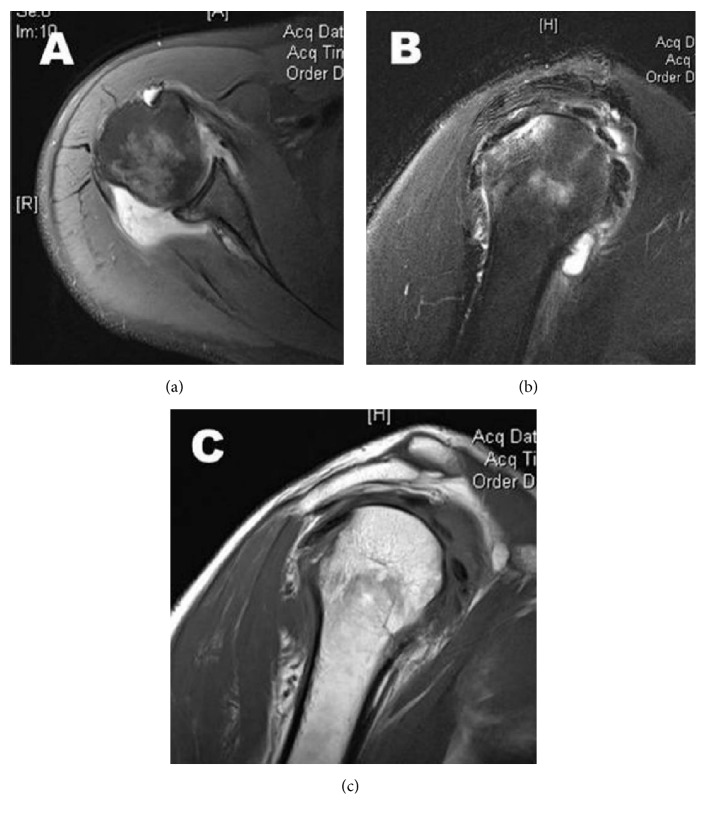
MRI of the right shoulder. (a) Axial proton density fat suppressed weighted image showing regions of low signal intensity within the joint effusion. (b) Sagittal proton density fat suppressed weighted image showing suppression of the signal intensity of the lesions, similar to subcutaneous fat. Bone erosion is present at the superior aspect of humeral head representing a Hill-Sachs fracture. (c) Sagittal T1-weighted image showing a large joint effusion and heterogeneous signal intensity within the periphery of the joint, suggestive of synovial hyperplasia.
